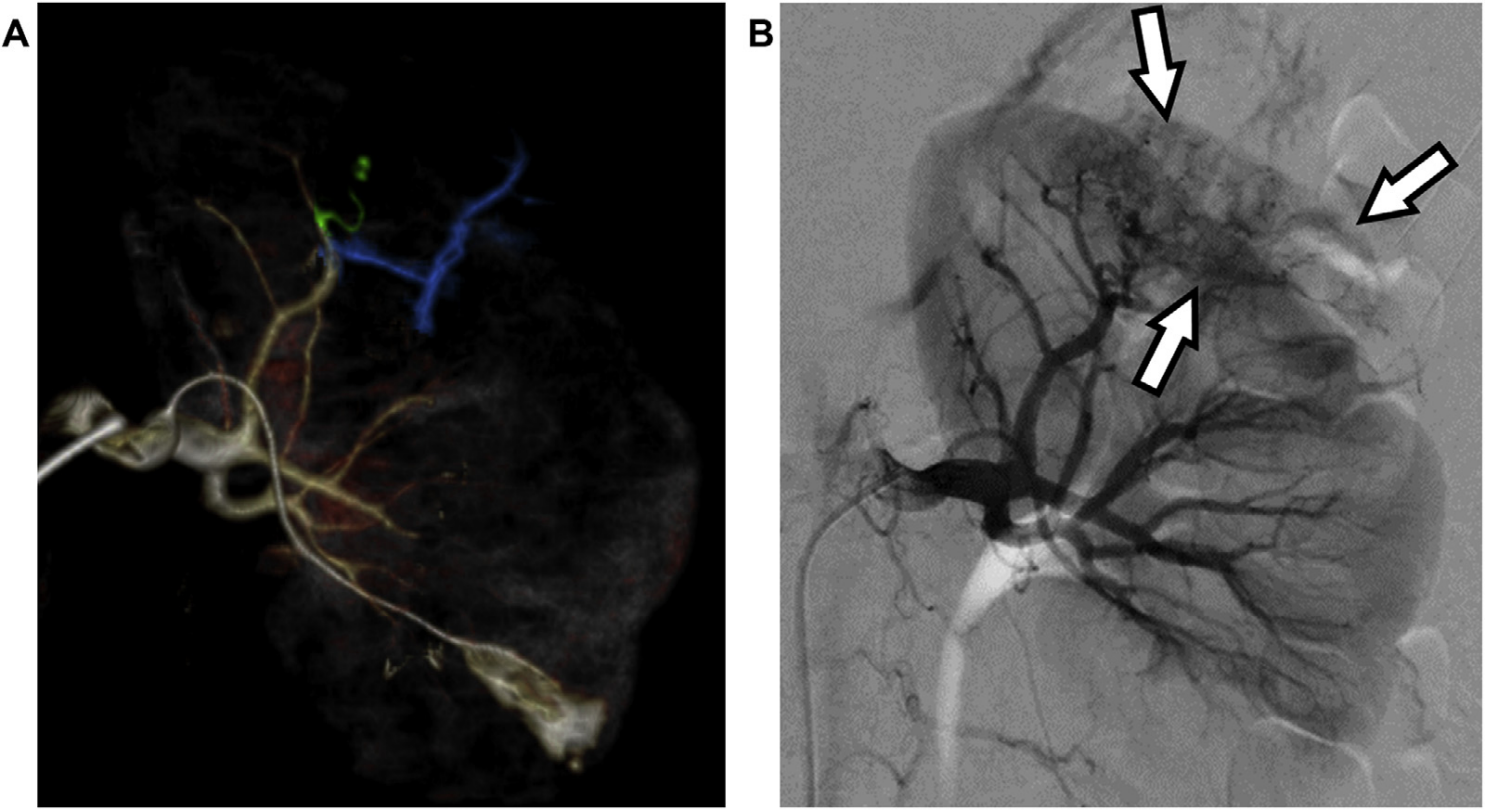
(A) VR image created from computed tomography-arteriography shows the feeding artery of the tumor (blue + yellowish green) and the branch contiguous to the aneurysm (yellowish green). (B) Pre-embolization left renal arteriography image shows the renal angiomyolipoma (arrows) and thin meandering feeding vessels inside the mass.
